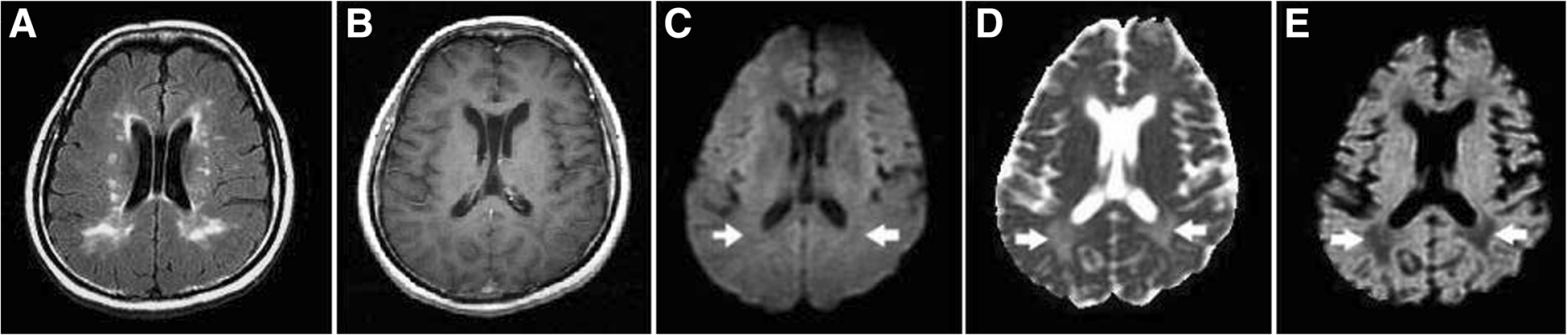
Type IVB lesions in a patient with MS. (A) Axial T2-FLAIR brain MRI shows multiple demyelinating lesions in the bilateral periventricular white matter. (B) All of the lesions show no enhancement on the CE T1WI. (C–E) Two of the lesions (arrows) in the posterior periventricular white matter are hypointense on the DWI, hyperintense on the ADC map, and hypointense on the eADC map, indicating chronic lesions with increased water diffusion due to severe tissue destruction.
